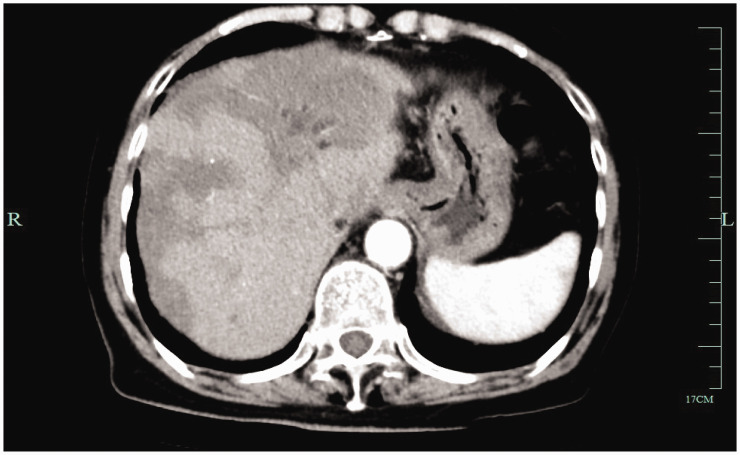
Enhanced computed tomography showing no tumor progression 3 months after starting anlotinib.
